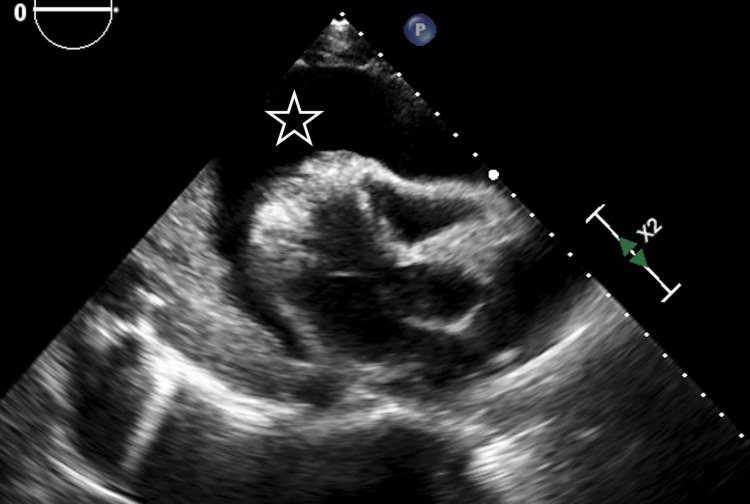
Transthoracic echocardiography (TTE) showing pericardial effusion (star)
